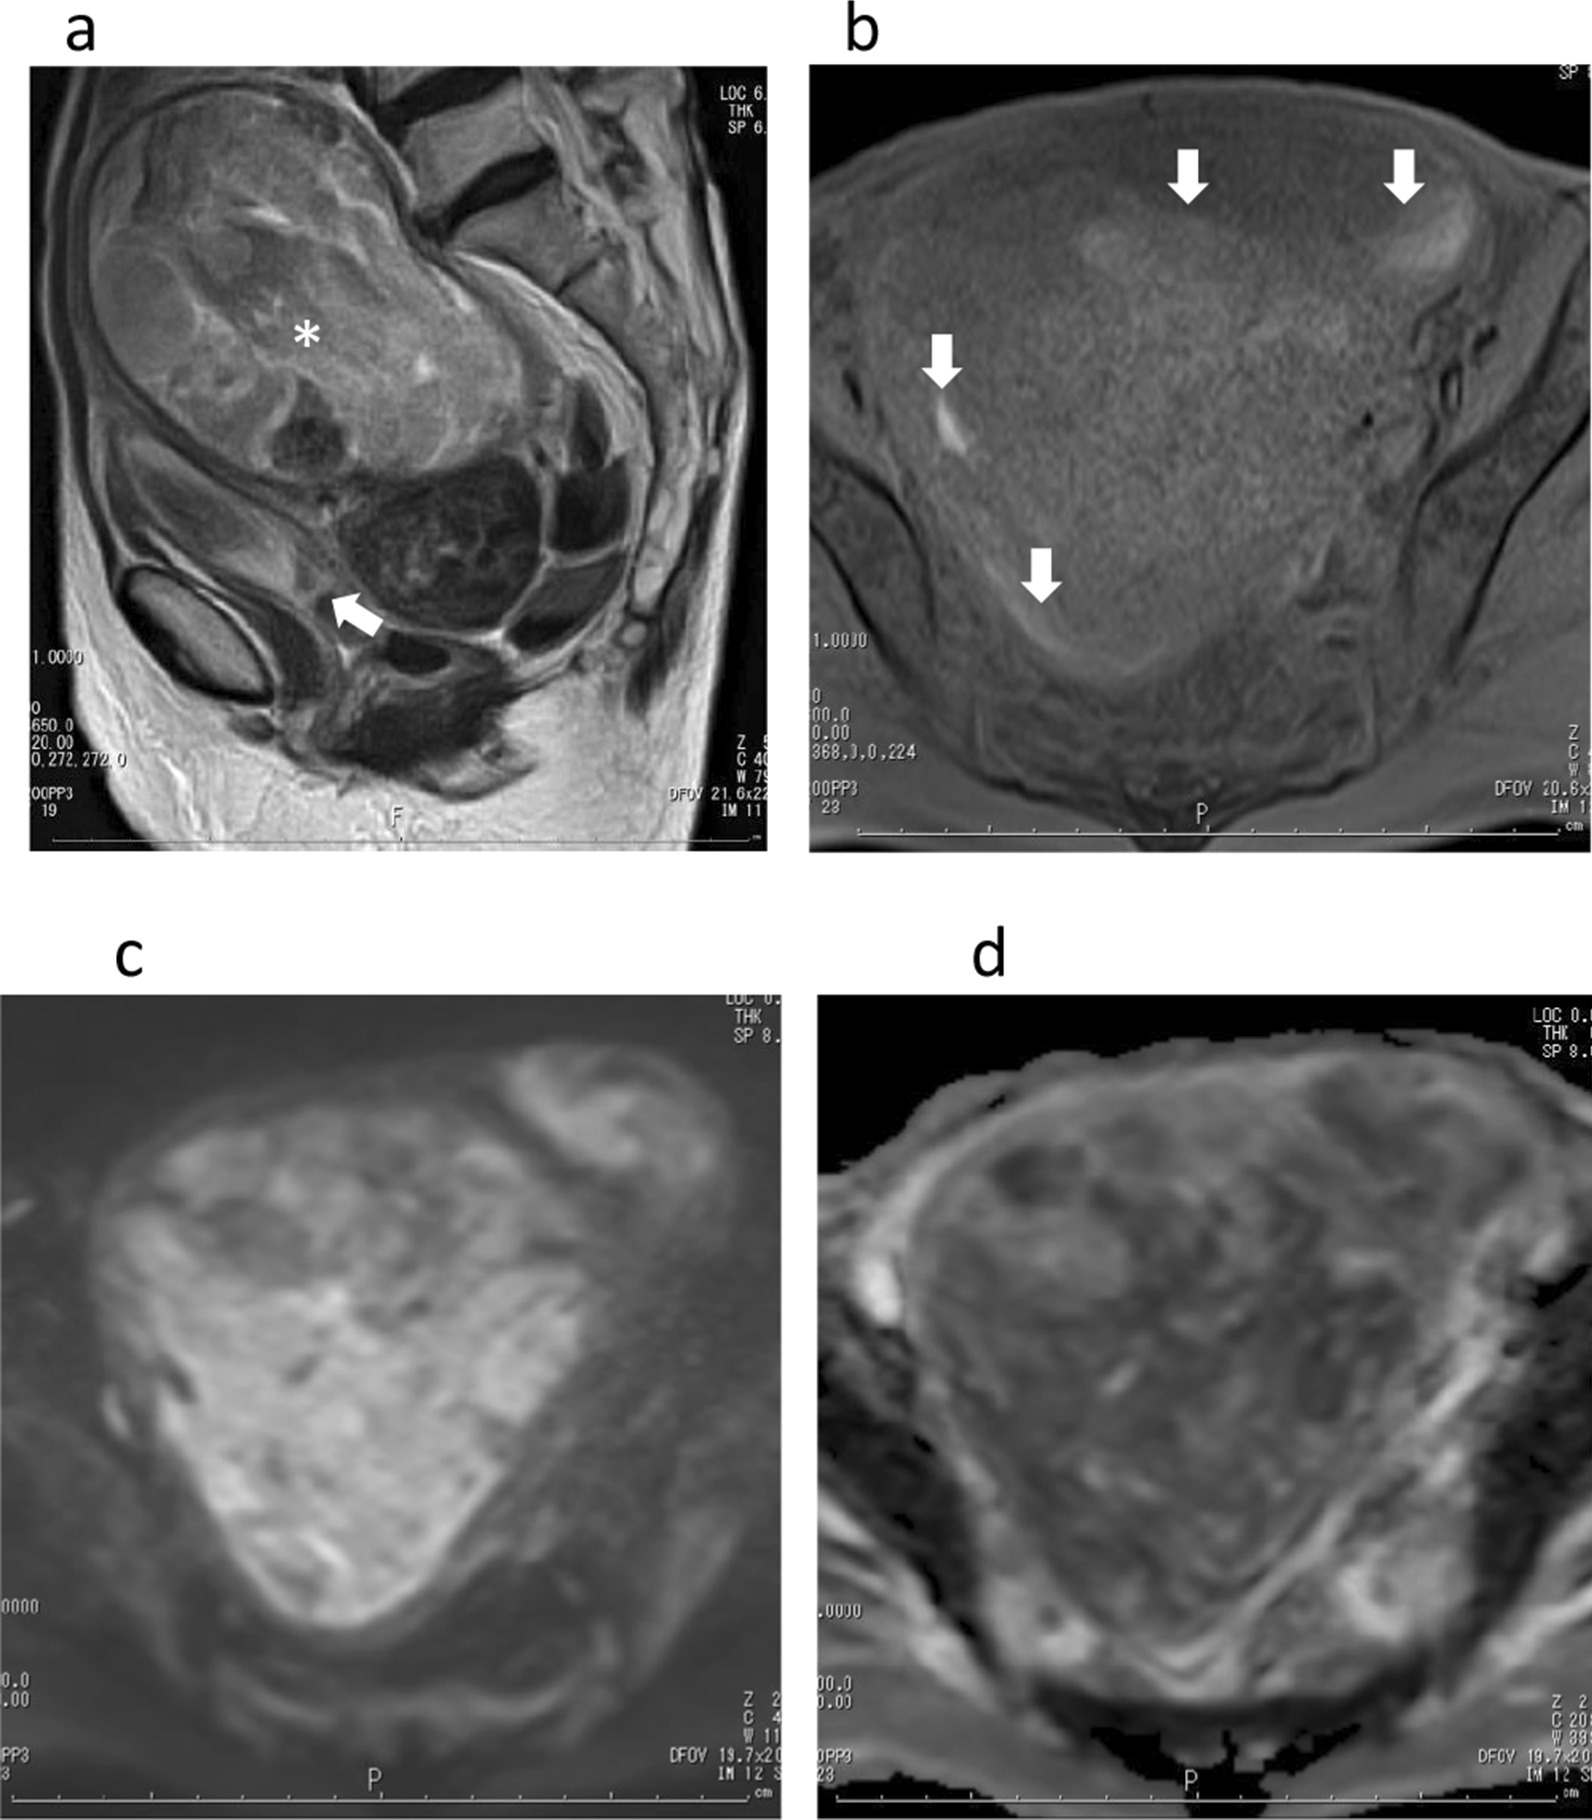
(a) Sagittal T2-weighted imaging (repetition time [TR]/echo time [TE], 4650/120 ms) showed a large, heterogeneous high-intensity mass without myometrial invasion expanding the uterine cavity (*) and extending into the cervical canal (arrow). (b) The mass showed low signal intensity with areas of slightly high signal intensity (arrows) on fat-suppressed T1-weighted imaging (TR/TE, 600/10 ms). (c) The mass showed high intensity on diffusion-weighted imaging (b = 1000 s/mm^2^, TR/TE, 7000/100 ms) and low intensity (1.26 × 10^−3^ mm^2^/s) on apparent diffusion coefficient maps (d)
